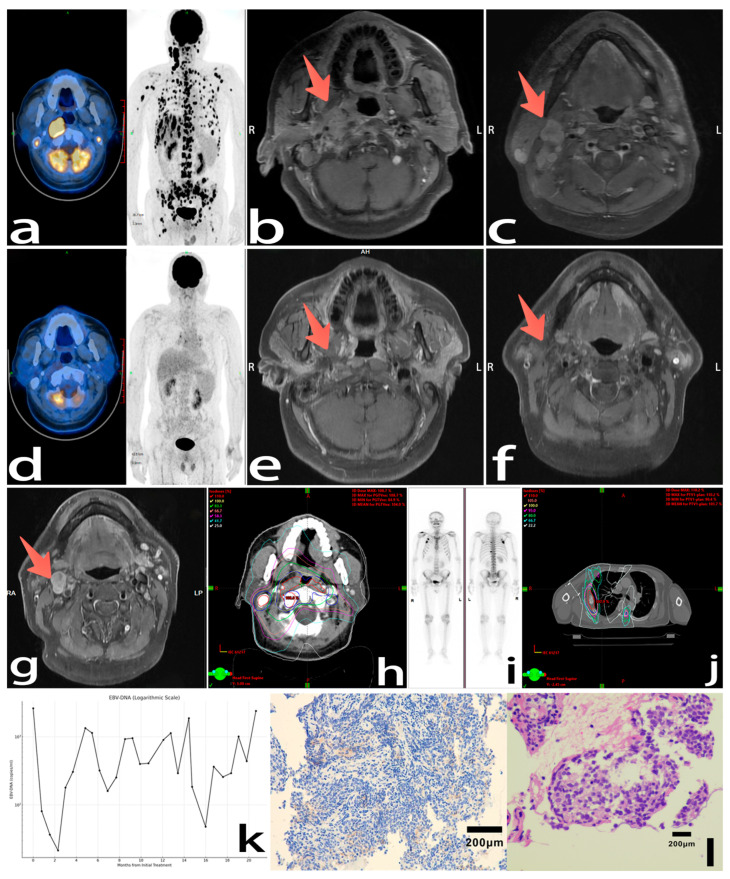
Initial diagnostic imaging: (a) Pre-treatment PET-CT demonstrated NPC with widespread metastatic involvement of bone and liver. (b,c) Pre-treatment MRI delineating nasopharyngeal primary and cervical nodal metastases. Post-first-line treatment evaluation: (d) post-six-cycle systemic therapy PET-CT showed metabolic resolution of primary and metastatic tumors (SUVmax 2.5 vs. baseline 22.6). (e,f) MRI-confirmed complete response (CR) with nasopharyngeal lesion and cervical lymph nodes. Post-second-line treatment evaluation: (g) Progressive right cervical nodal enlargement with necrotic rim sign. (h) Radiation dosimetry map for primary site palliative RT. (i) SPECT-confirmed osseous progression. (j) Metastatic rib lesion radiation planning. (k) EBV-DNA load fluctuations: EBV-DNA copies dropped remarkably but soon rose and remained relatively high until the last follow-up. (l) Pathological results suggested an undifferentiated non-keratinizing carcinoma.
